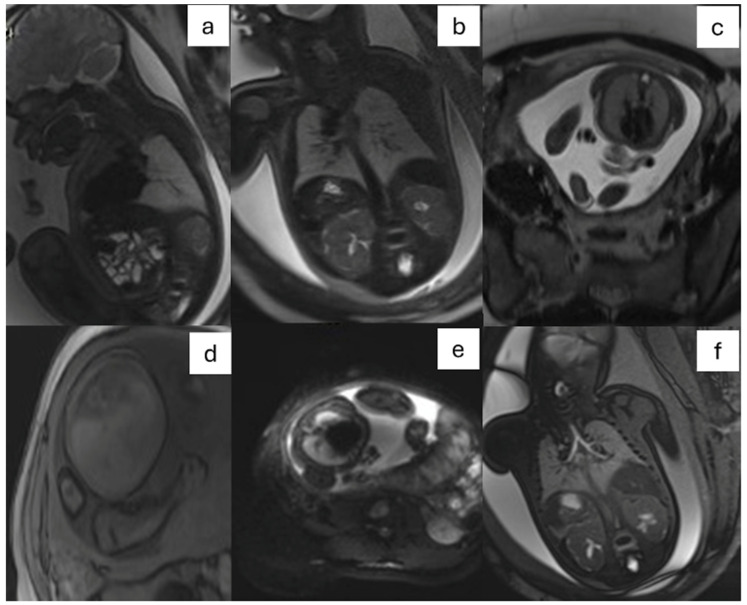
Fetal chest MRI protocol: T2-weighted single-shot fast spin echo in sagittal, coronal, and axial (a–c) planes; T1-weighted three-dimensional (3D) GRE on axial plane (d); DWI EPI (e) on axial plane; balanced steady-state-free precession on coronal plane (f). DWI: diffusion-weighted imaging; EPI: echo planar imaging; GRE: gradient echo; MRI: magnetic resonance imaging.
